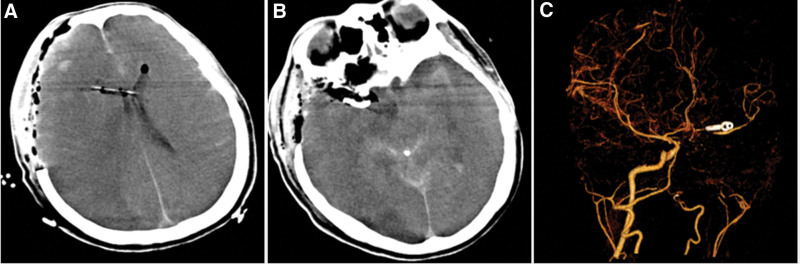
(A and B) Repeat head CT showed massive cerebral infarction in the right cerebral hemisphere after aneurysm clipping surgery. (C) CTA showed right internal carotid artery was disappeared, suggesting occlusion completely.
